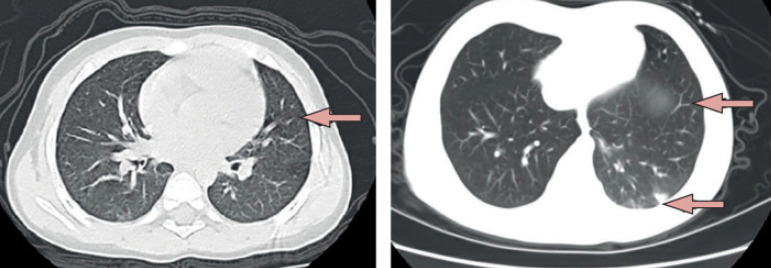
Chest computed tomography (CT) scans from two paediatric patients with coronavirus disease 2019[5]. Left: CT of 10-year-old boy showing multiple opacities in lower lobes of both lungs (arrow). Right: CT of 1.5-year-old girl showing multiple ground-glass opacities with a big patchy opacity in the right lung (arrows).
